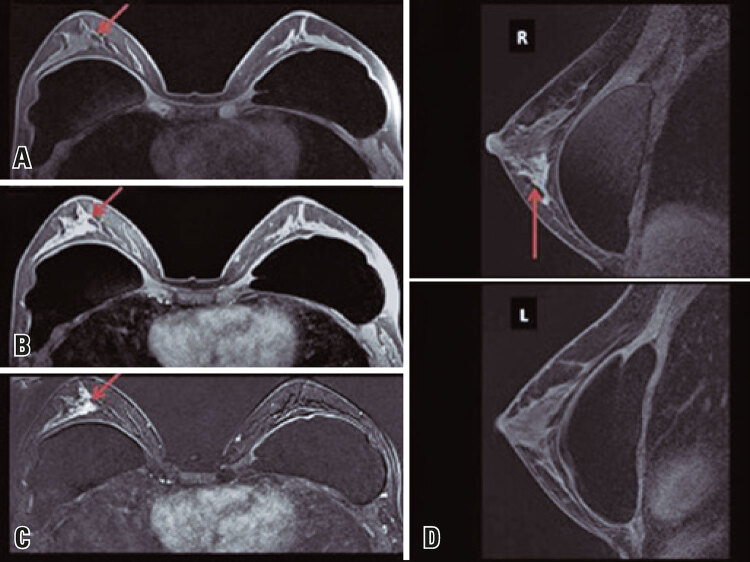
Female, 41 years old, family history of breast cancer. Breast magnetic resonance imaging revealed a focal area of heterogeneous and asymmetric enhancement measuring 3.3 x 4.0 x 2.3cm at the union of the lower quadrants of the right breast (red arrows), classified as BI-RADS 4. (A) Fat-suppressed pre-contrast T1 image; (B) Second post-contrast T1 sequence; (C) Subtraction image (post-contrast - pre-contrast); (D) Sagittal reconstruction of post-contrast image
